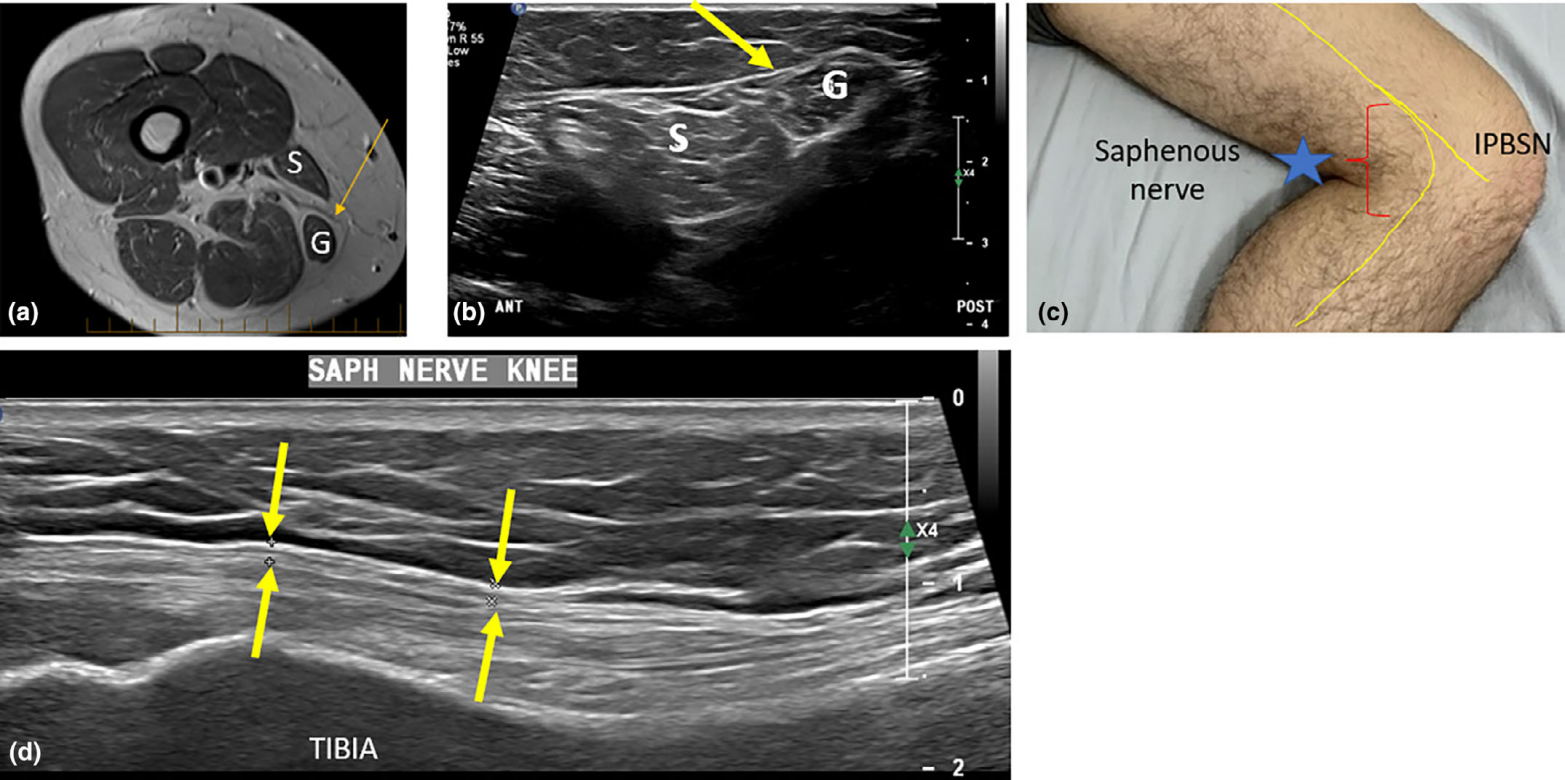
Saphenous nerve at the knee. (a). Axial magnetic resonance (MR) image of the distal thigh, demonstrating the saphenous nerve (orange arrow) passing between the sartorius (S) and gracilis (G) muscles to become superficially located. (b). A short‐axis sonographic image indicating the position of the sartorius and gracilis muscles and the saphenous nerve (yellow arrow) passing between these, where it pierces the crural fascia to become superficially located. (c). The medial knee showing the region in which the saphenous nerve pierces the fascia (red brackets and blue star), posterior to the infrapatellar branch of the saphenous nerve (IPBSN). (d). Sonographic long‐axis image of the saphenous nerve (between yellow arrows) once it has become superficial (approximately 1 mm diameter at this point).
